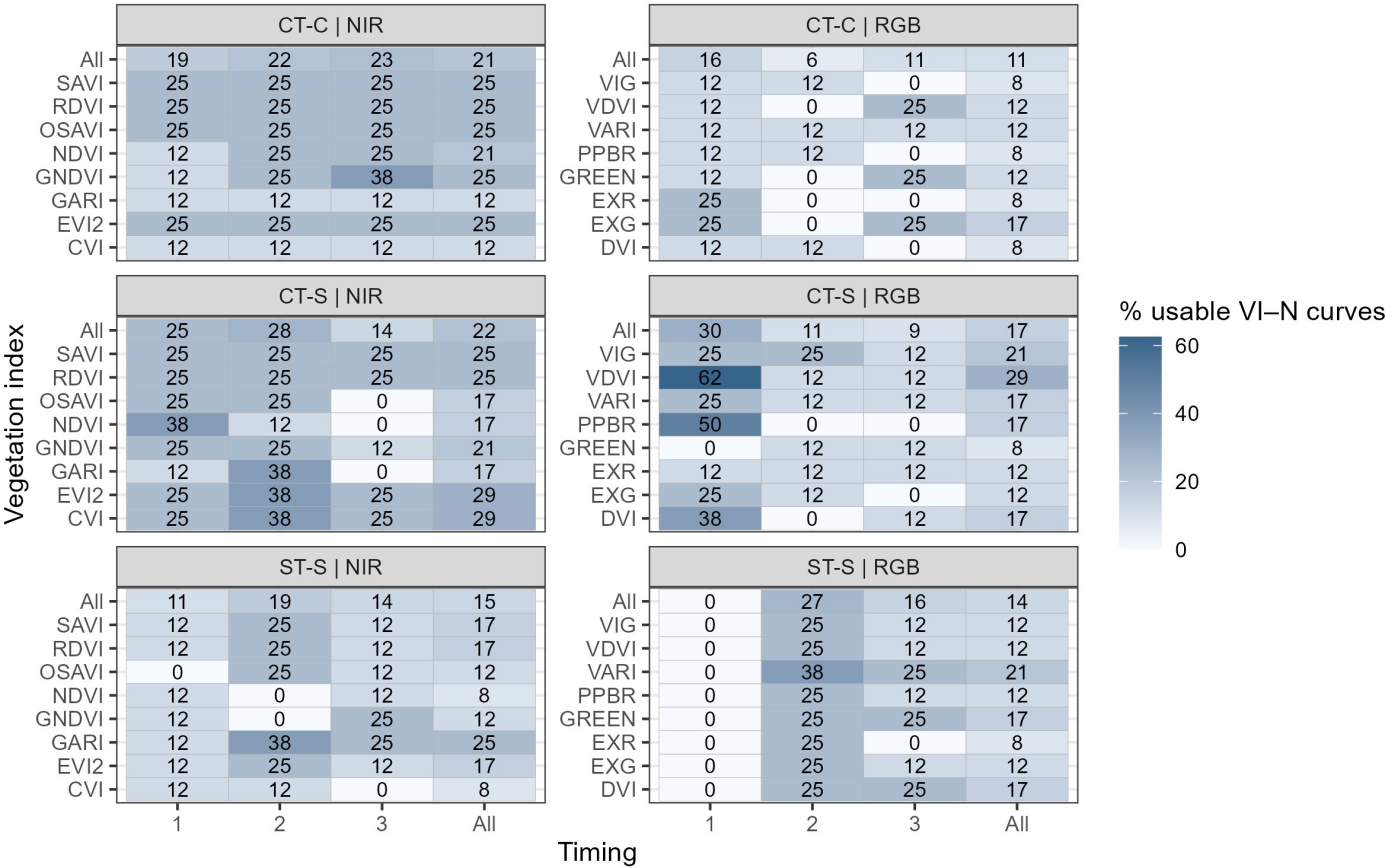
Percentage of VI–N response curves retained after applying model selection criteria, separated by field trial. Field names indicate tillage (ST, strip-till; CT, conventional tillage) and previous crop (C, corn; S, soybean). Values are shown by vegetation index (rows) and image timing (columns; 1, V7 and V8; 2, V10 and V11; 3, V15 and V16), including overall counts across timings and VIs (“All”). Panels are separated by VI type: near-infrared (NIR)-based indices (left) and RGB-based indices (right). VIs names and formulas are described in Table 4.
